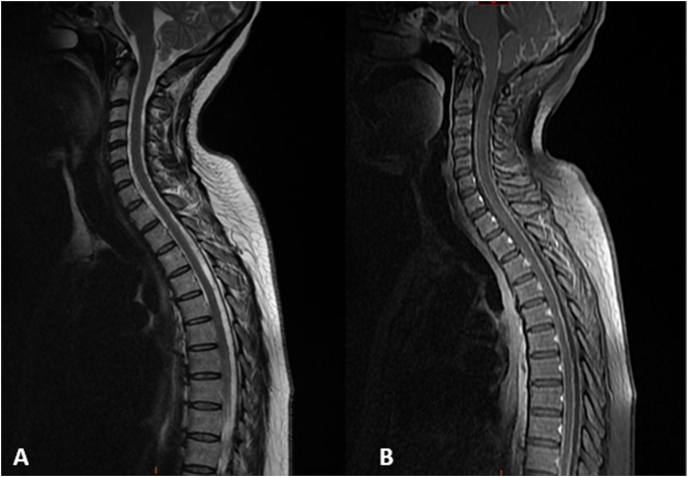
Sagittal T2 (A) and T1 C+ (B) spine MRI showing discrete T2 hyperintensities with contrast enhancement along the posterior column of the cervical spinal cord.
